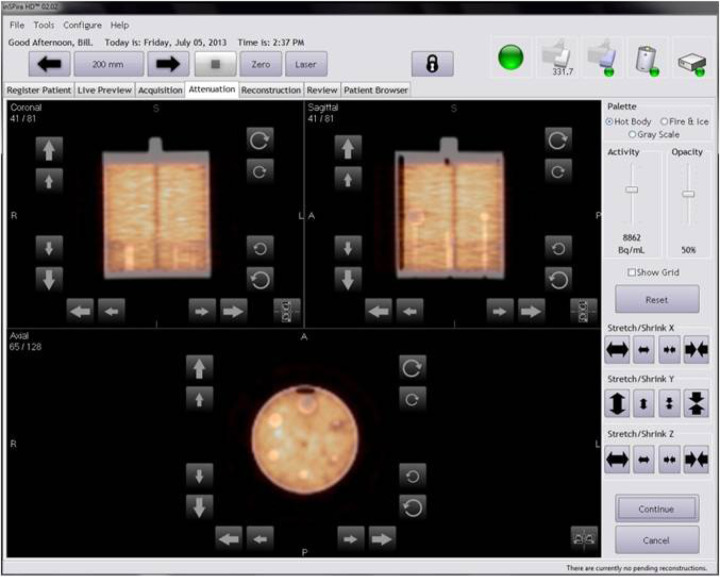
The interface for registering SPECT and CT images. Software allows for translation, rotation, scaling, and flipping about all three axes. The color lookup table (i.e., temperature map) for the SPECT scan and the blending between CT and SPECT data can also be altered to improve visualization of the alignment.
